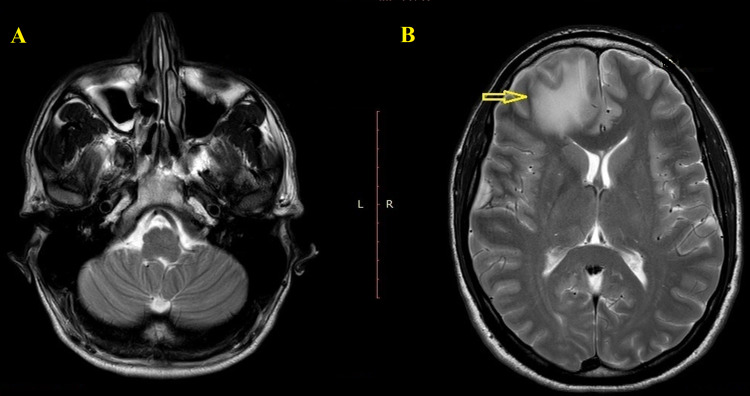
Six months postoperative MRI.
